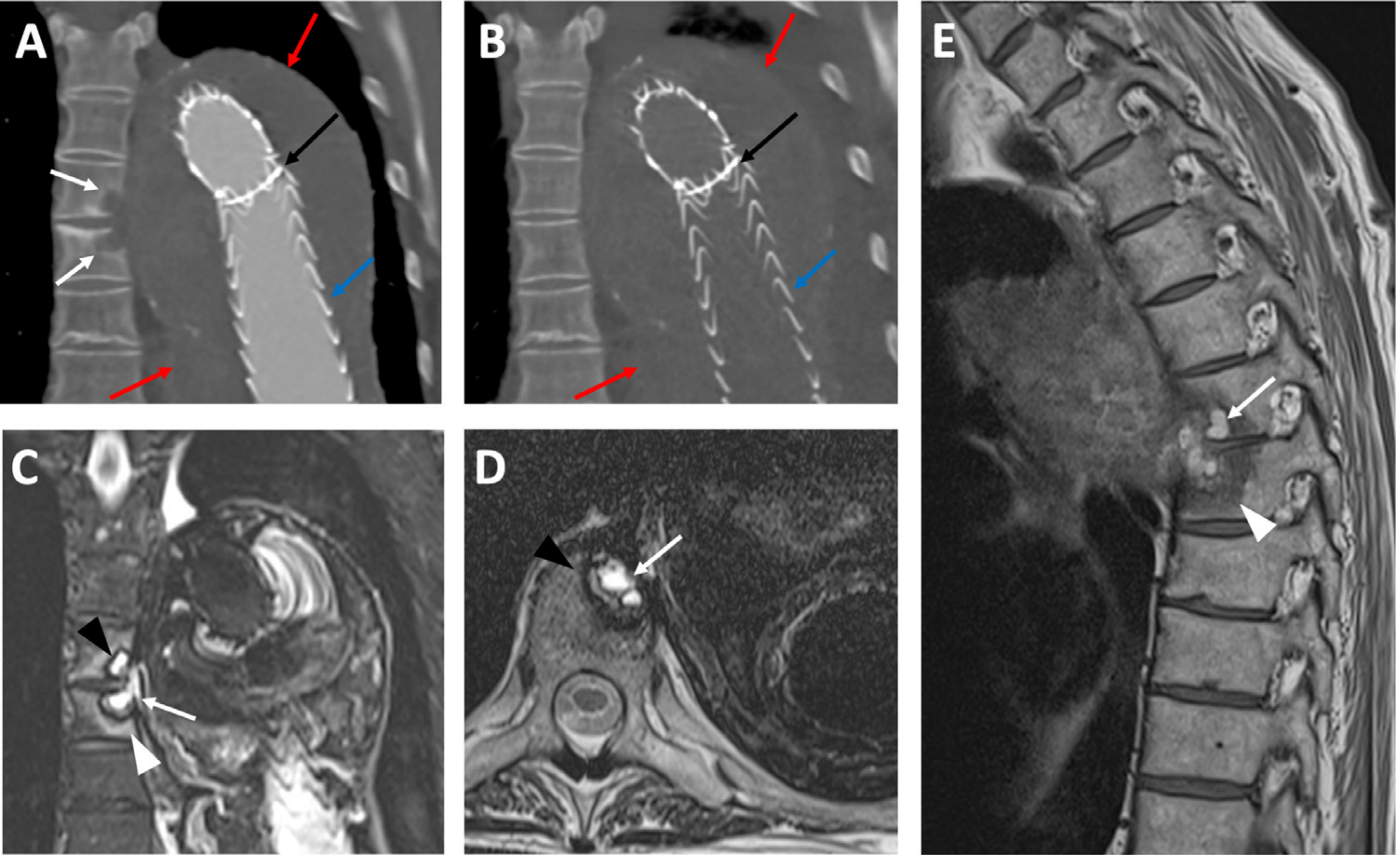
Coronal reconstruction of a regular follow-up CTA after surgical and endovascular treatment of a thoraco-abdominal aneurysm (A). Stent grafts placed into the thoraco-abdominal aneurysm (blue arrow), overlapping areas of 2 stent grafts (black arrow). New erosions of the sixth and seventh thoracic vertebra are noted (white arrows). Non-contrast CT Chest (B) 2 months prior to A shows no vertebral erosions. MRI performed 3 weeks after CT of image B (C-E): T2 TIRM-weighted coronal image (C) shows the known vertebral erosion (white arrow), with a T2- and T1-weighted hyperintense Signal (D, E), corresponding to internal hemorrhage. The erosion shows a T2-/T1-weighted hypointense rim (black arrowhead), corresponding to hemosiderin deposits (D). Surrounding T2-weighted hyperintense and T1w-hypointense edema in both vertebrae (white arrowhead) (C-E).
